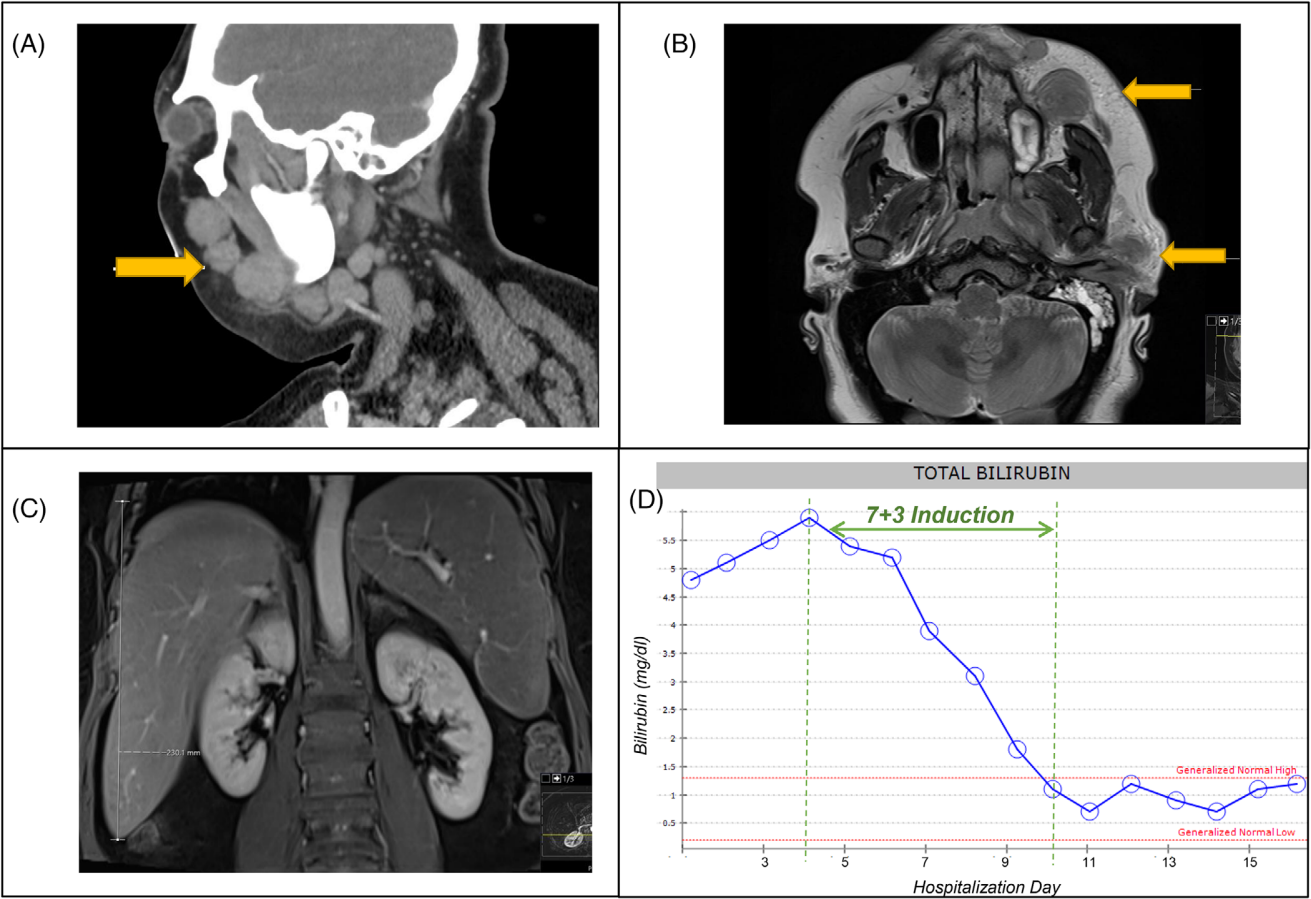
(A, B) Computed tomography and magnetic resonance (MR) imaging confirmed multiple soft tissue masses in the left face and neck, including a 2.7 cm mass anterior to the maxilla, 3.1 cm left parotid mass, and 3.3 cm mass along the lateral margin of the mandible. (C) Abdominal MR imaging showed hepatomegaly measuring 23 cm and splenomegaly measuring 17 cm (craniocaudal dimensions) but no discrete masses or biliary obstruction. (D) Trend in total bilirubin showing response to induction, with a steady decrease continuing until normalization by day 7.
